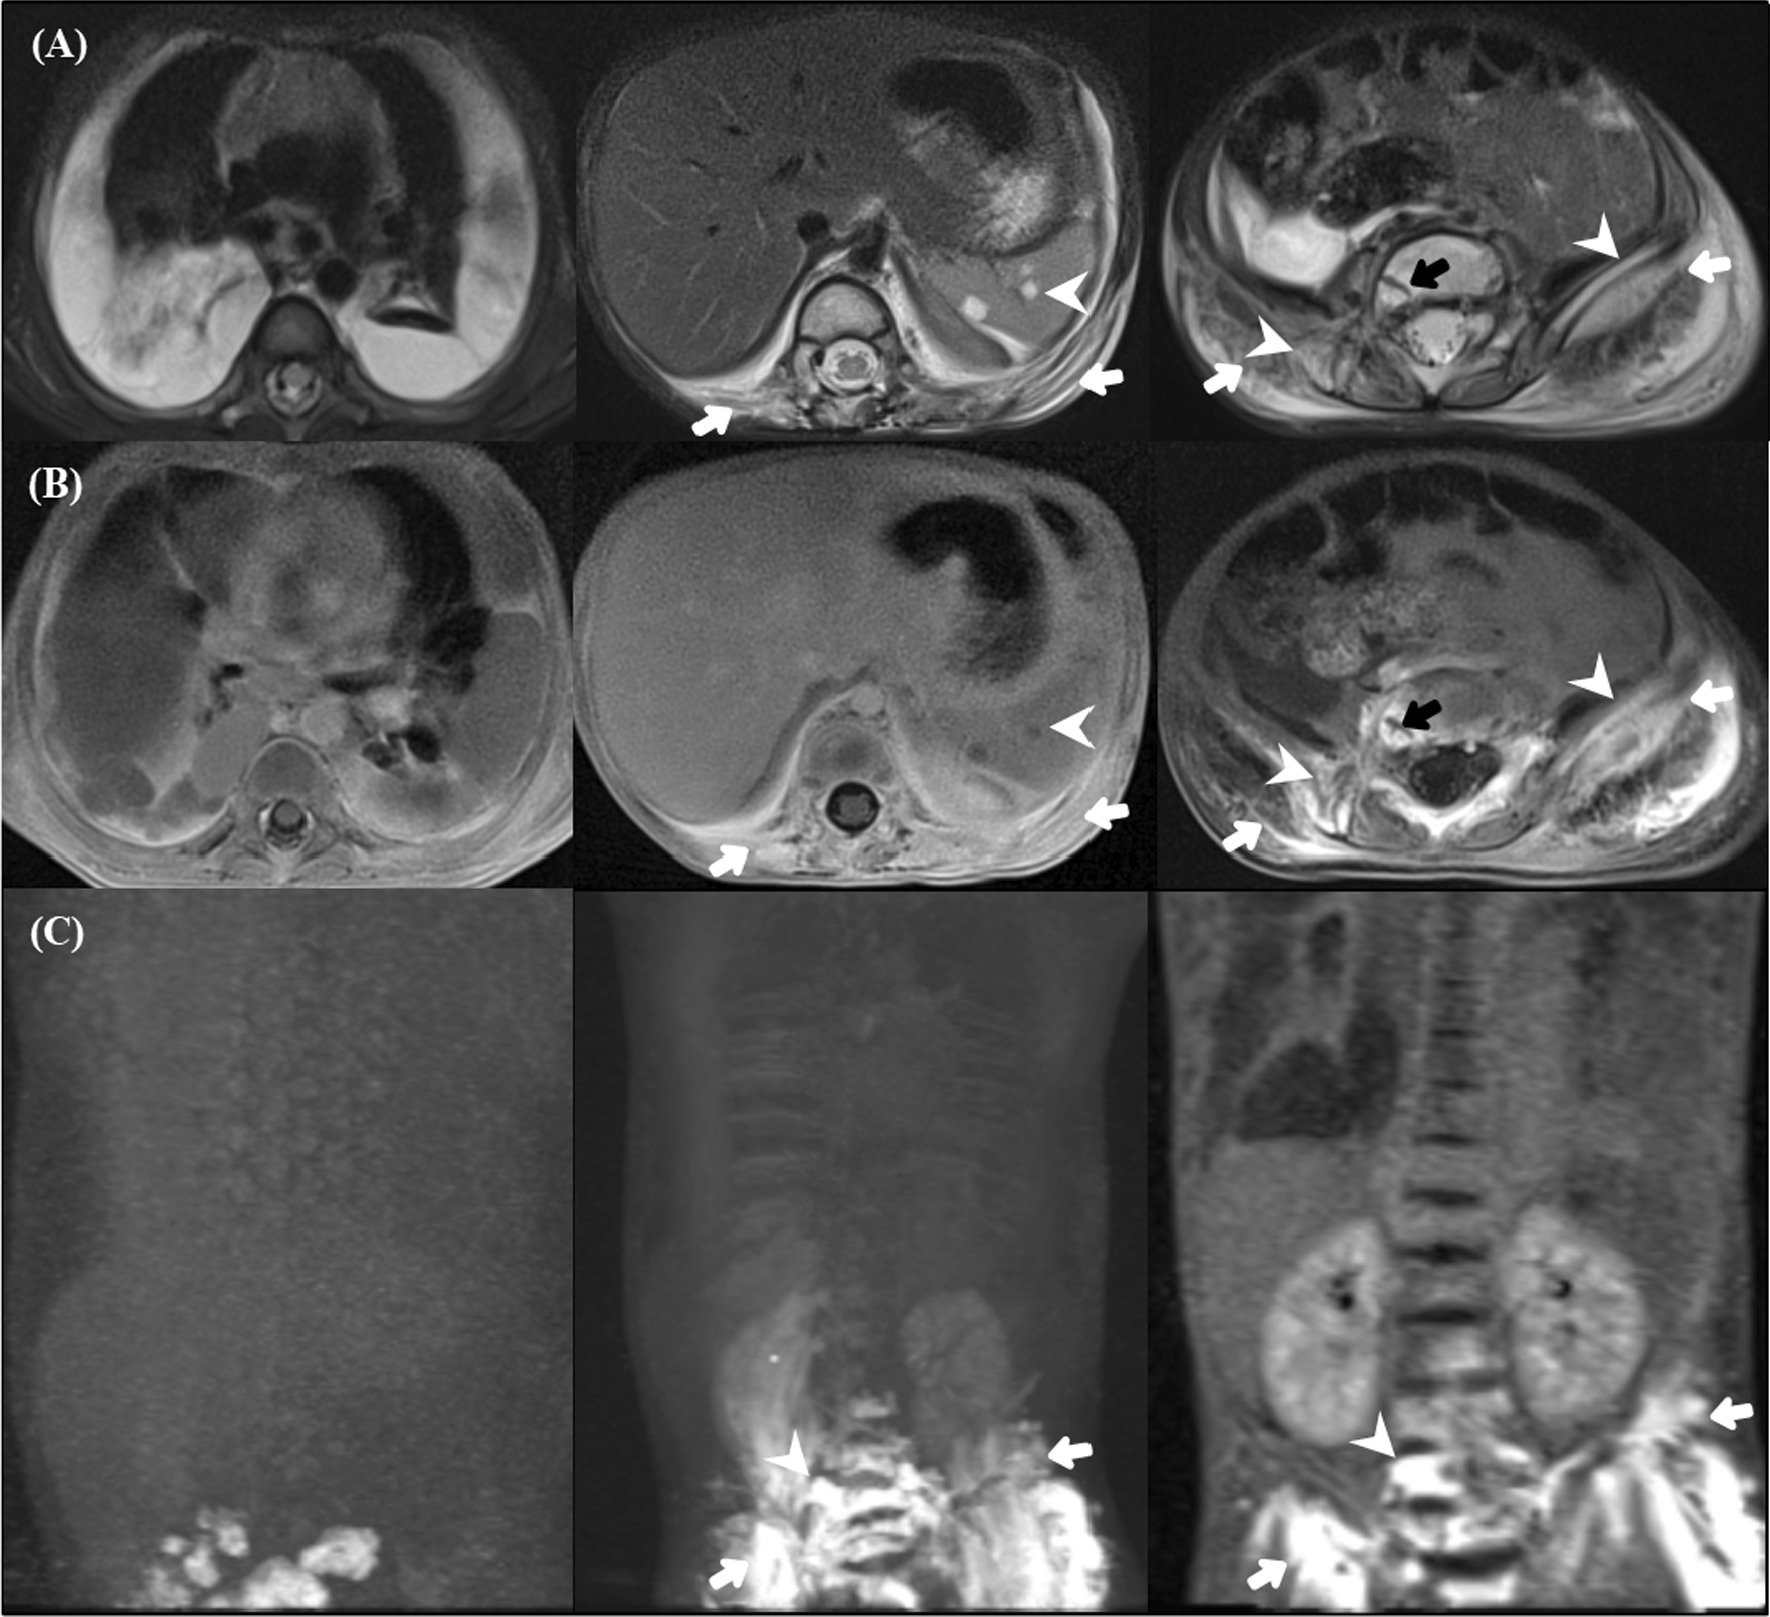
A 3-year-old female with Gorham–Stout disease. A 3-year-old female patient diagnosed with Gorham‒Stout disease presented with asymmetric buttock volume. Dynamic contrast-enhanced magnetic lymphangiography (DCMRL) consisted of axial T2-weighted image (T2WI), axial/coronal contrast-enhanced T1-weighted image (T1WI), and maximum intensity projection (MIP) reconstruction images. A There is a large volume of pleural effusion (first column), small volume of ascites, splenic cysts (second column, arrowhead), soft-tissue infiltration (second and third columns, white arrows), and bone lesions (third column, arrowheads) on T2WI. B There is bony enhancement in the ileum (third column, arrowheads) and vertebrae (black arrow), and soft-tissue thickening with enhancement (second and third columns, arrows). C MIP showing an abundantly enhanced retroperitoneal lymphatic system and soft tissue (arrows) and enhanced vertebrae (arrowheads), but the cisterna chyli and thoracic duct were not visible
